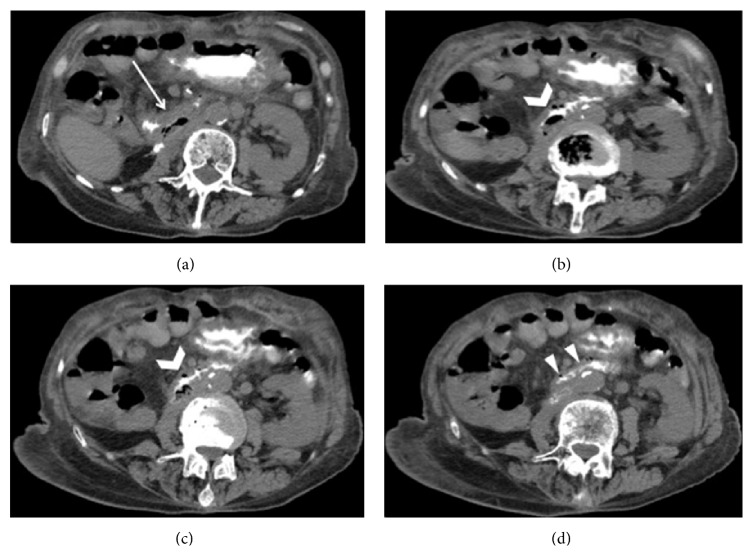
Transverse abdominal unenhanced CT scan acquired after oral administration of a water-soluble contrast agent. The images show the collapse of the second part of the duodenum (white arrow (a)) and the presence of an air bubble collection (open arrow (b) and (c)) (35 × 35 × 15 mm), located between the posterior wall of the duodenum and the collapsed IVC graft. The transaxial CT images of the lower tract of the duodenum demonstrate the trouble of assessing attenuation differences between IVC graft and luminal contrast agent (arrowheads (d)).
